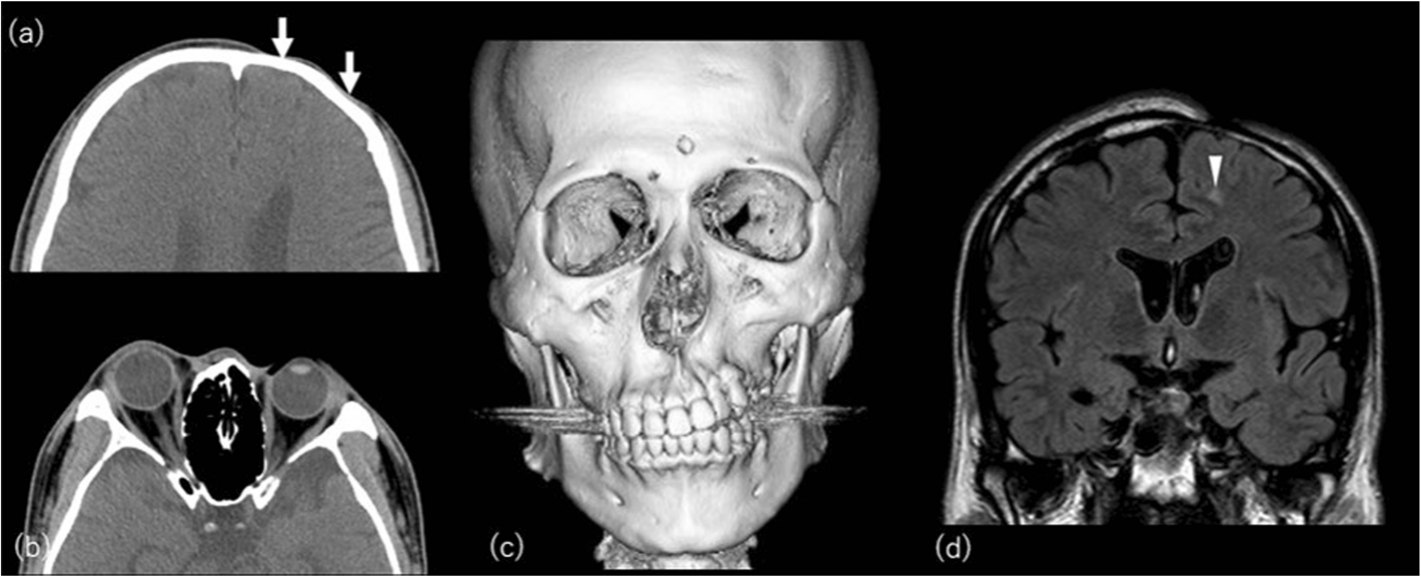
A 29-year-old man with Parry-Romberg syndrome. a Axial CT images show asymmetrically decreased subcutaneous fat on the left side (arrows). b Hypoplasty of the left orbit is also evident. c Volume-rendered three-dimensional reformation image of facial bones highlights bony asymmetry of the face. There is a perceivable asymmetry of the maxillary bone as well. d Coronal FLAIR image shows subcortical high signal intensity area (arrowhead)
